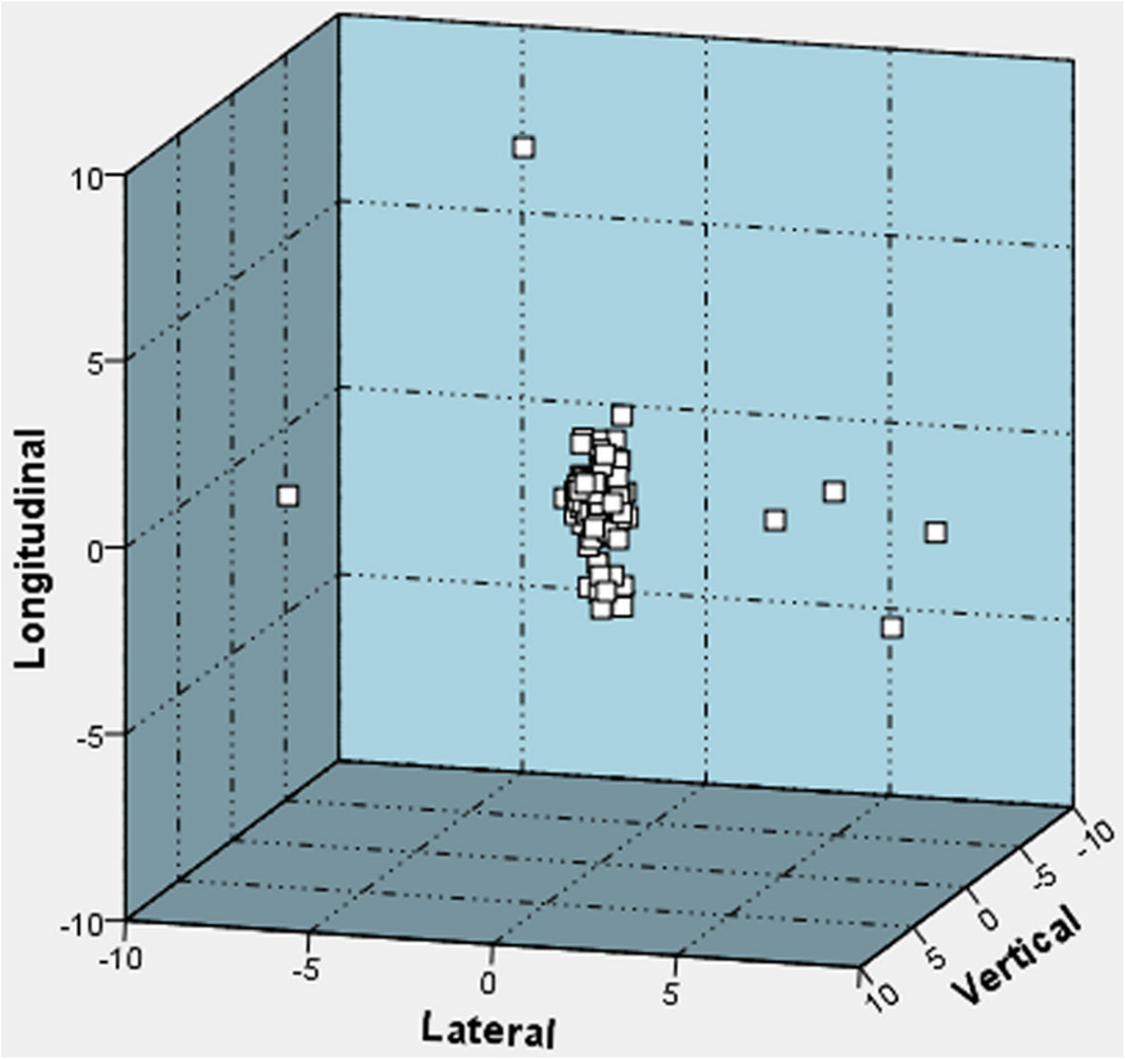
Online adjustments after cone beam computed tomography scans with low pressure foil [cm]
